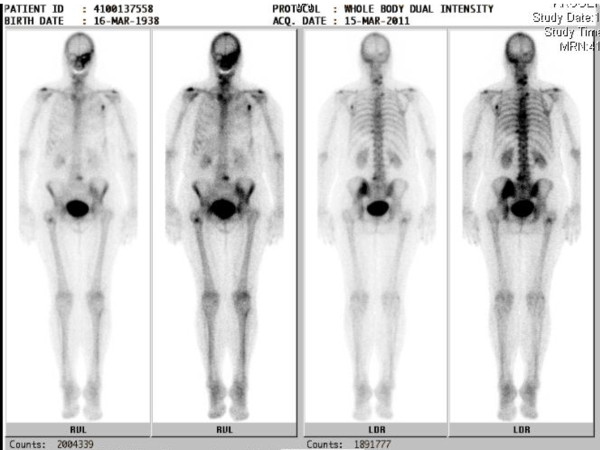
Technetium-Bone scintigraphy reveals multiple osseous lesions in the skeleton suspicious for metastases of the breast cancer.
